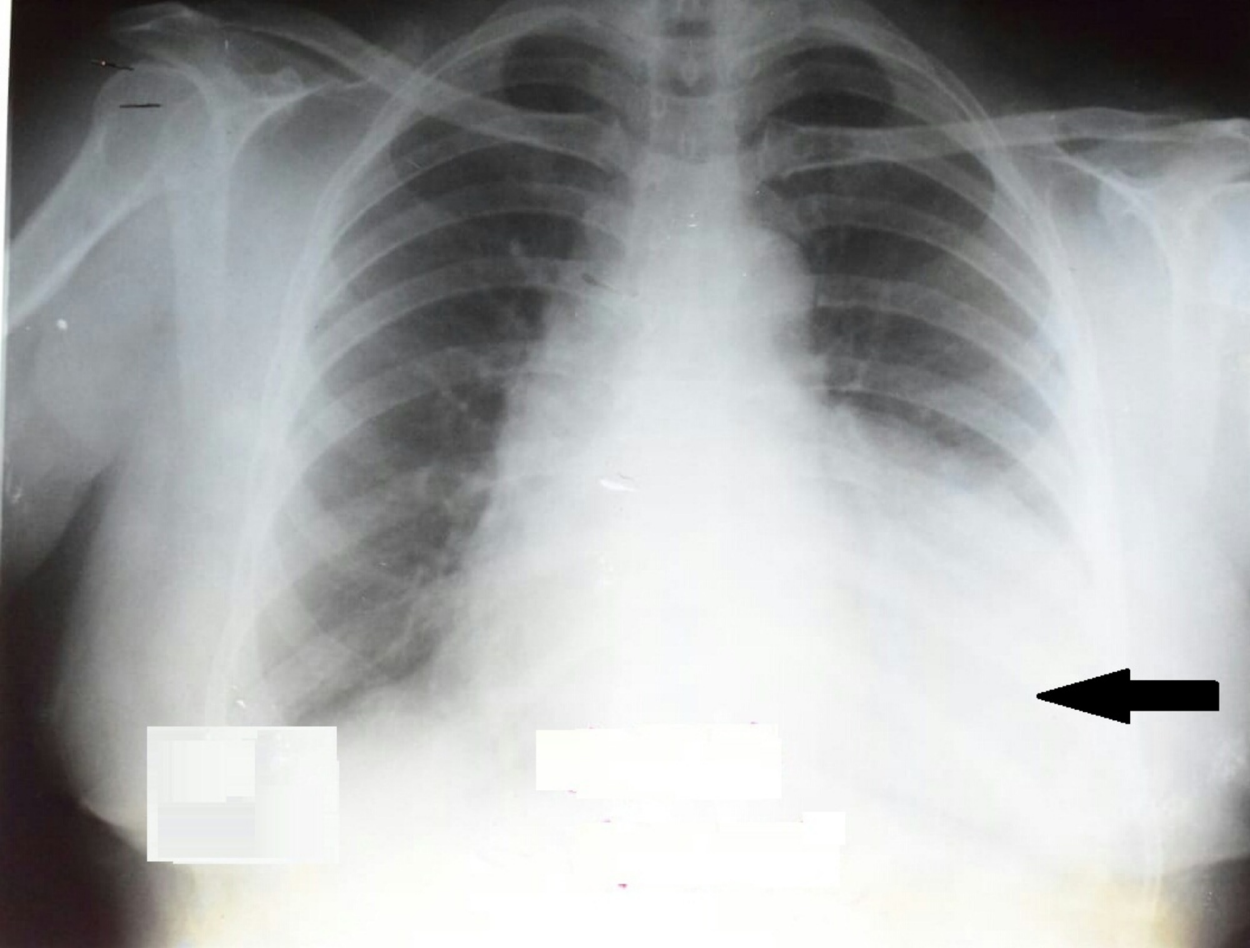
Posteroanterior chest radiograph showing a non-homogeneous opacity involving the left lingual segment and left lung lower lobe (marked by an arrow). Loss of silhouetting of the left heart border is also evident.
